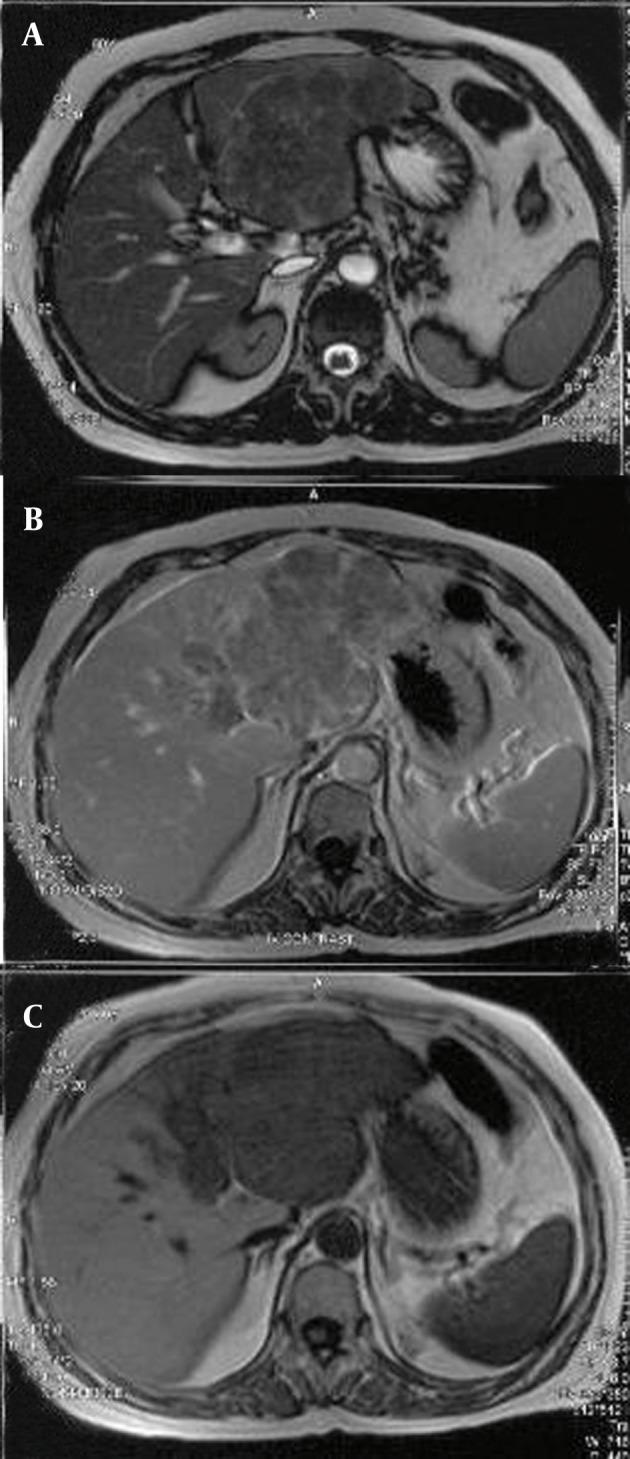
MRI in HCC A, Axial T2-W image shows heterogeneous high signal area, pseudocapsule and central hyposignal area in favor of necrosis; B, T1-W image after gadolinium injection shows enhancement of the mentioned mass in the left lobe; C, Axial T1-W shows hyposignal mass in the left lobe.
